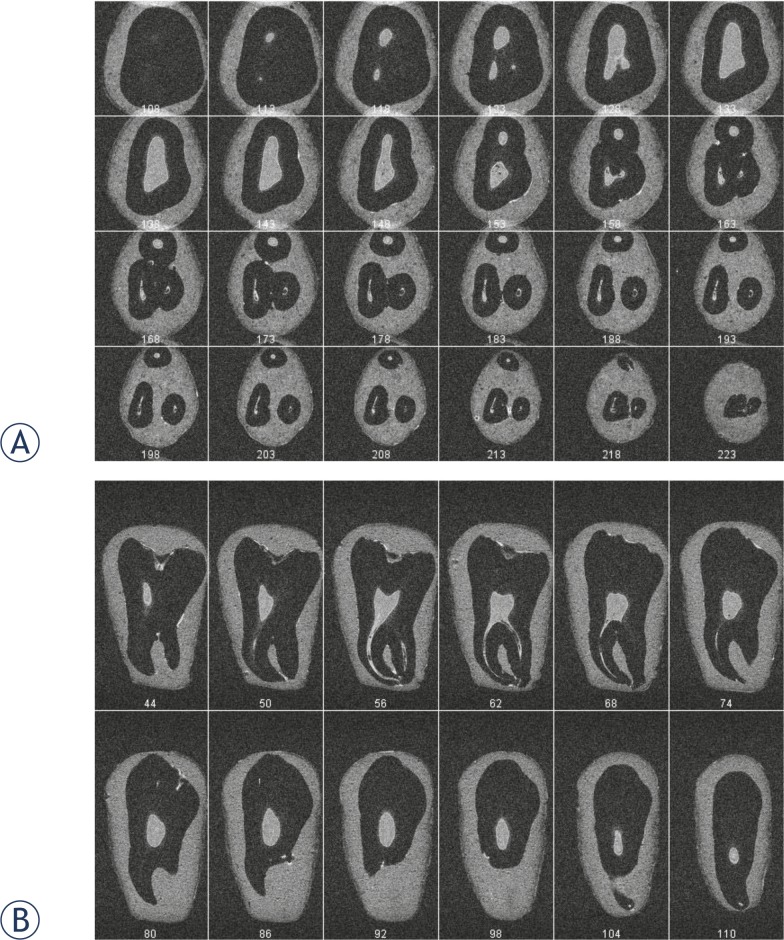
Images of consecutive 24 horizontal (A) and 24 vertical (B) slices across the dental pulp in Figure 2. The images are subsets of the 3D T1-weighed MR image of the pulp acquired using imaging matrix 256 × 128 × 128 and isotropic image resolution of 100 μm; numbers at the bottom of each image are slice indices. The pulp anatomy in horizontal plane is visualised from the coronal parts to the apical foramina. In horizontal slices, shape and volume of the pulp chamber as well as the number and shape of root canals are presented. The cross section of the mesio-buccal and distal canal is spherical in contrary to the mesio-lingual canal, which is more oval and kidney-shaped. A precise inspection of the mesio-lingual canal in slice 65 shows that its cross section is elongated from mesial to distal direction and that it could consist of two canals. Vertical slices are convenient for tracking of the course of single root canals.
